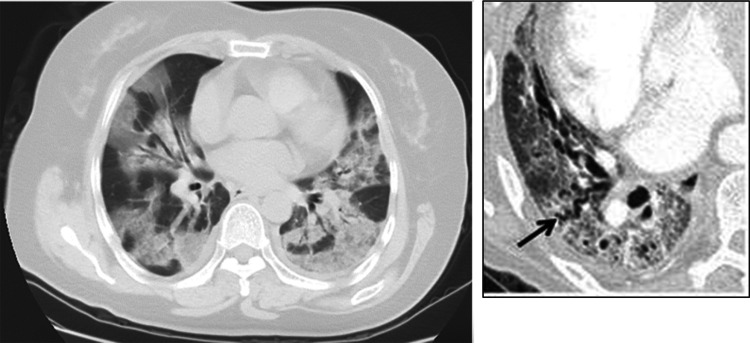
CT chest images showing dense consolidation Left-hand side image: Axial chest CT showing the bilateral and extensive distribution of GGOs and consolidation. Right-hand side image: CT image displaying crazy-paving pattern (black arrow). Image source: Reference no. [7,18]
